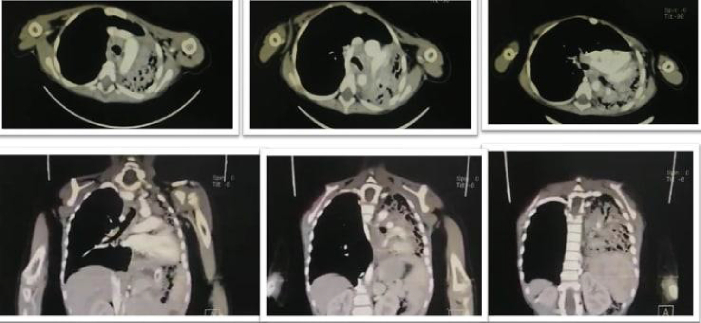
The CT scan images show atelectasis in the left lung with the destruction of the lung tissue (shows wide infiltrations /alveolar pattern/ in the left lung).
